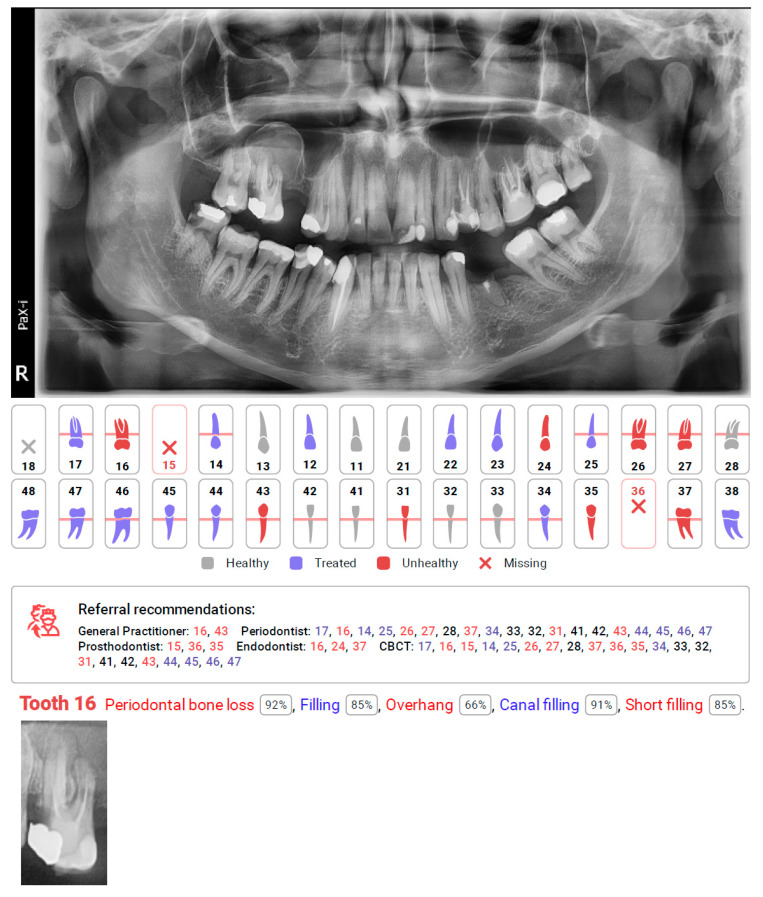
Diagnocat report, with missing detection of cyst in the right maxillary sinus in the region of tooth 16 and automating caption of tooth with detected other pathologies. Referral recommendations suggest additional CBCT diagnosis for this tooth as well as consultation with an endodontist.
